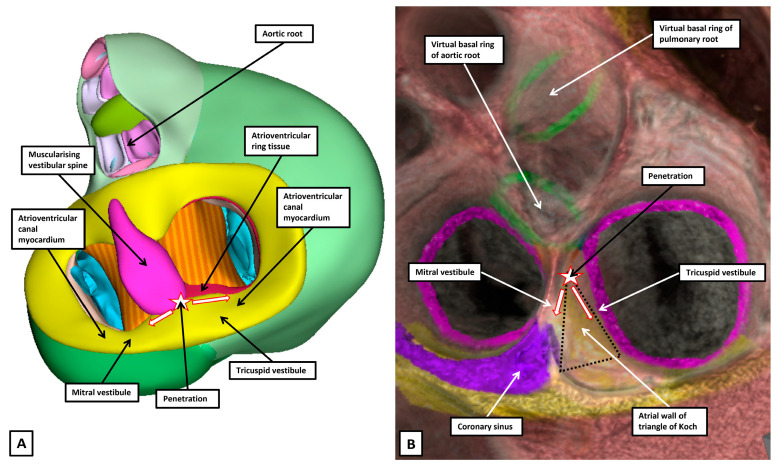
The panels show the major changes required to transform the arrangement at the end of the embryonic period of development (panel A) to the definitive arrangement (panel B). (A) Panel A is a reconstruction of an embryonic heart at 8 weeks of development. There has been no expansion of the atrioventricular junctions to produce the inferior pyramidal space, and the aortic root has yet to be “wedged” between the superior parts of the right and left atrioventricular junctions. Note how the primary ring emerges from beneath the inferior atrioventricular cushion to enter the tricuspid vestibule (See also Figure 11B). Both vestibules, however, are derived from atrioventricular canal myocardium, which is slowly conducting. (B) Panel B shows a virtual dissection of a computed tomographic dataset from a living patient. It is the expansion of the junctions that produces the triangle of Koch and the inferior pyramidal space. Note how the virtual basal ring of the aortic root has become wedged between the superior components of the junctions.
